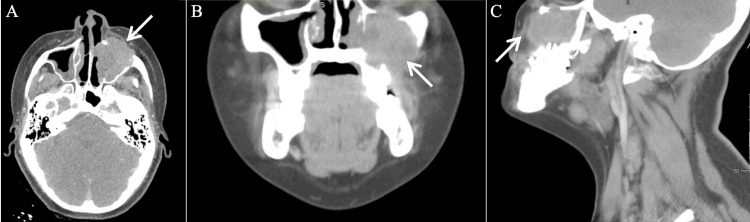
Initial axial (A), coronal (B), and sagittal (C) CT (neck) images demonstrating a left maxillary sinus mass with erosion of the anterior and posterior maxillary wall and left orbital floor with the rim intact
